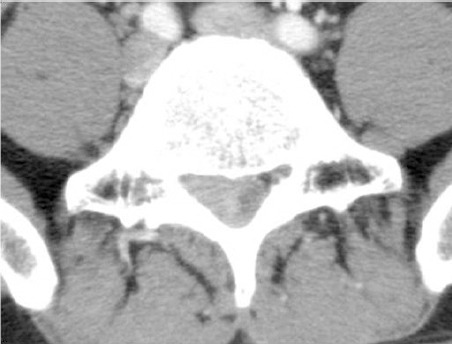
A subtly enhancing mass-like lesion in the right epidural space without bony destruction, observed on the lumbar spine CT scan
